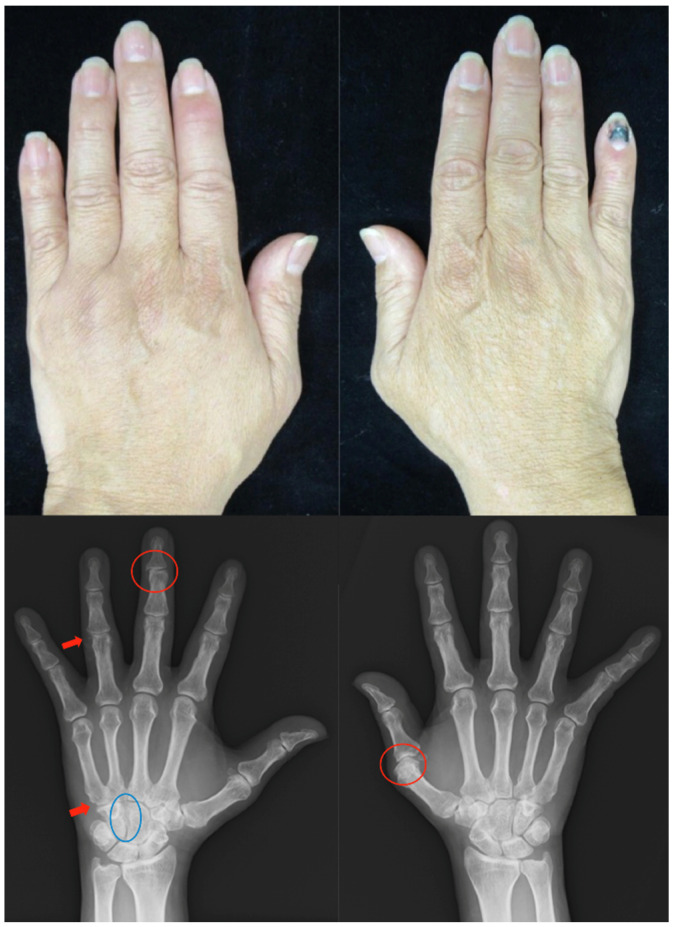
Clinical photographs and radiographs of the hands in case 4. Swelling, pain, and redness are noted in the left second and right fifth joints. There is bone proliferation at the left third DIP and right first MCP joints due to PsA (red circle), marginal erosion in the left fifth proximal metacarpal and fourth PIP joints (red arrow), and joint space narrowing in the left carpal bone (blue circle). MCP, metacarpophalangeal; PIP, peripheral interphalangeal; DIP, distal interphalangeal.
